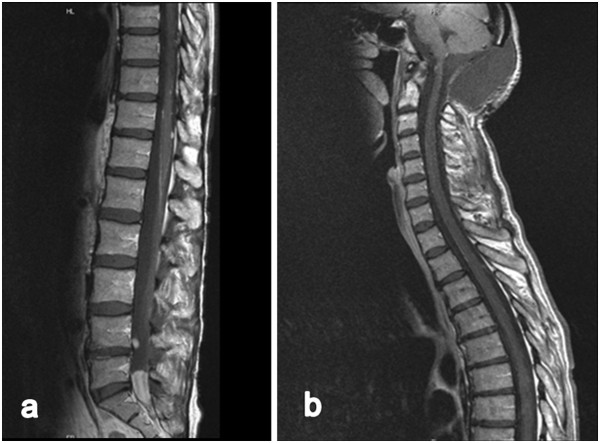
Sagittal T1-weighted magnetic resonance images enhanced with gadopentetate dimeglumine demonstrating spinal tumor located dorsal of lumbar vertebral body L 4 and a large tumor manifestation dorsal L5 to S1 (a) of approximately 4.0 × 1.2 cm in the sagittal view accompanied by multiple small enhancements in the range of the whole spinal cord (a, b).
